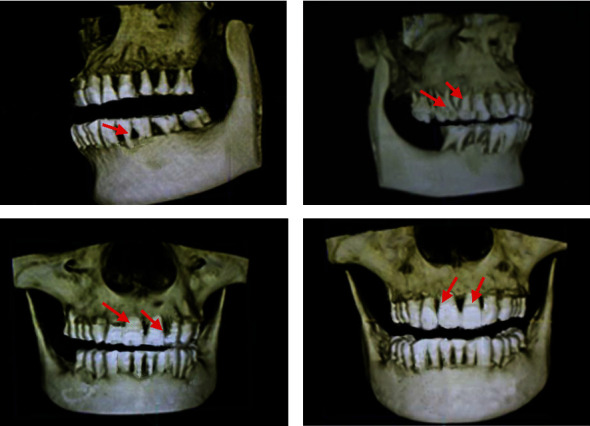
Three-dimensional CT image of severe periodontitis alveolar bone.
